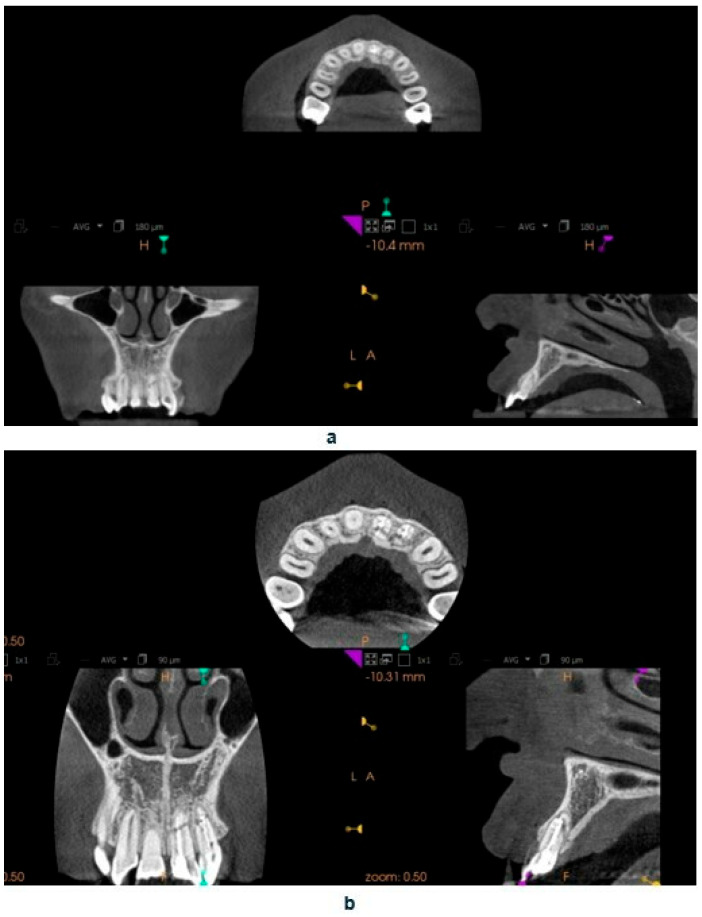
CBCT images presenting tooth no. 22: (a) before treatment, (b) after treatment.
